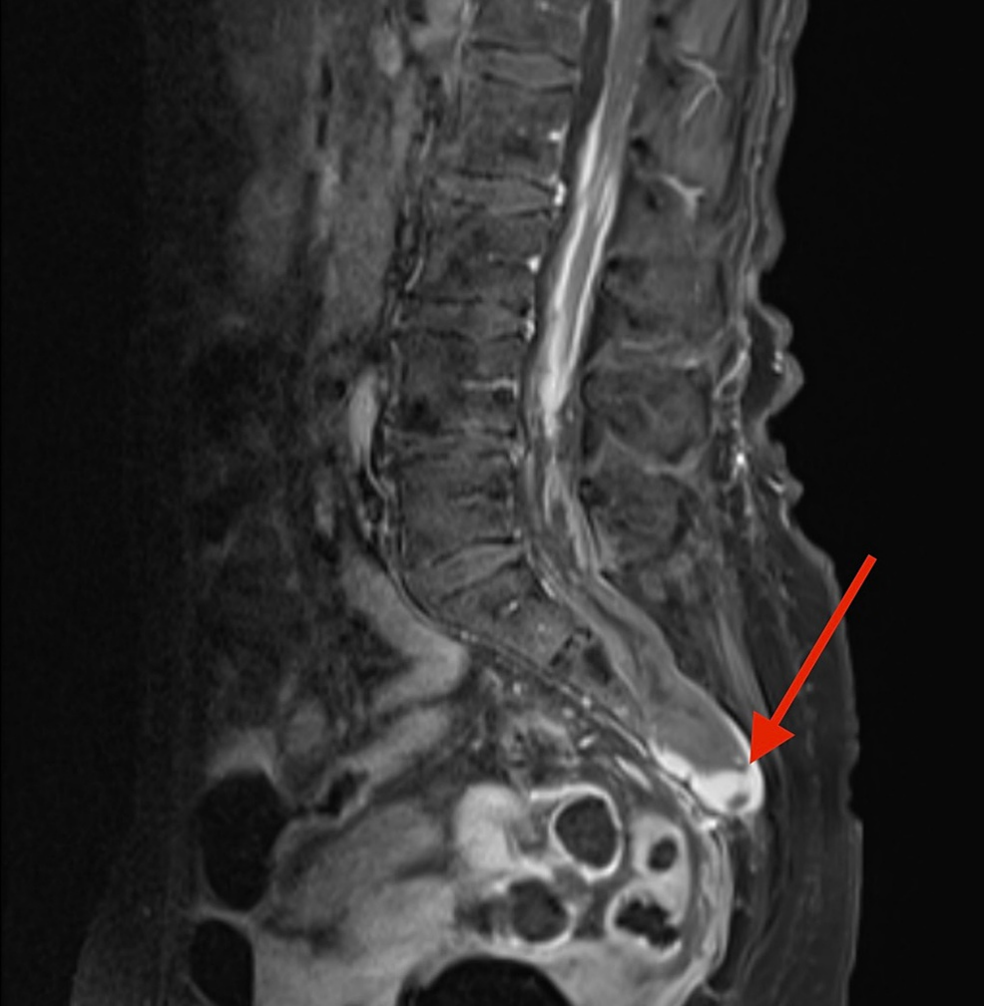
Sagittal T1 -weighted MRI with contrast revealing a high signal intensity 3 cm cystic lesion in the lumbosacral spine at S3-S4. Red arrow points to the lesion.
